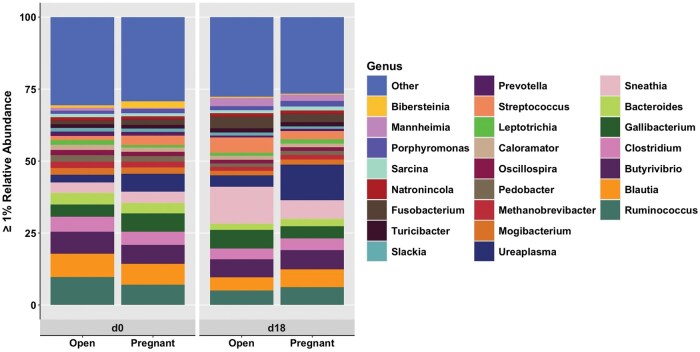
Genera greater than 1% relative abundance between days (d0 and d18) and pregnancy status (Open and Pregnant). Relative abundance as a percent is shown on the y-axis with pregnancy status within days shown on the x-axis. Differing colors represent different genera found in greater than 1% relative abundance within the vagina. Genera less than 1% relative abundance are combined into the “Other” category. Pregnancy status was determined by transrectal ultrasonography on d32 post-artificial insemination.
